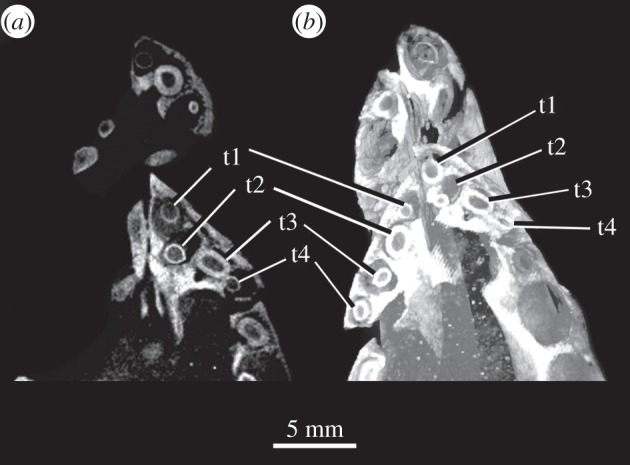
Euparkeria capensis* SAM-PK-6047A premaxillary teeth as (*a*) CT slice and (*b*) volume reconstruction from CT data. t, tooth.
